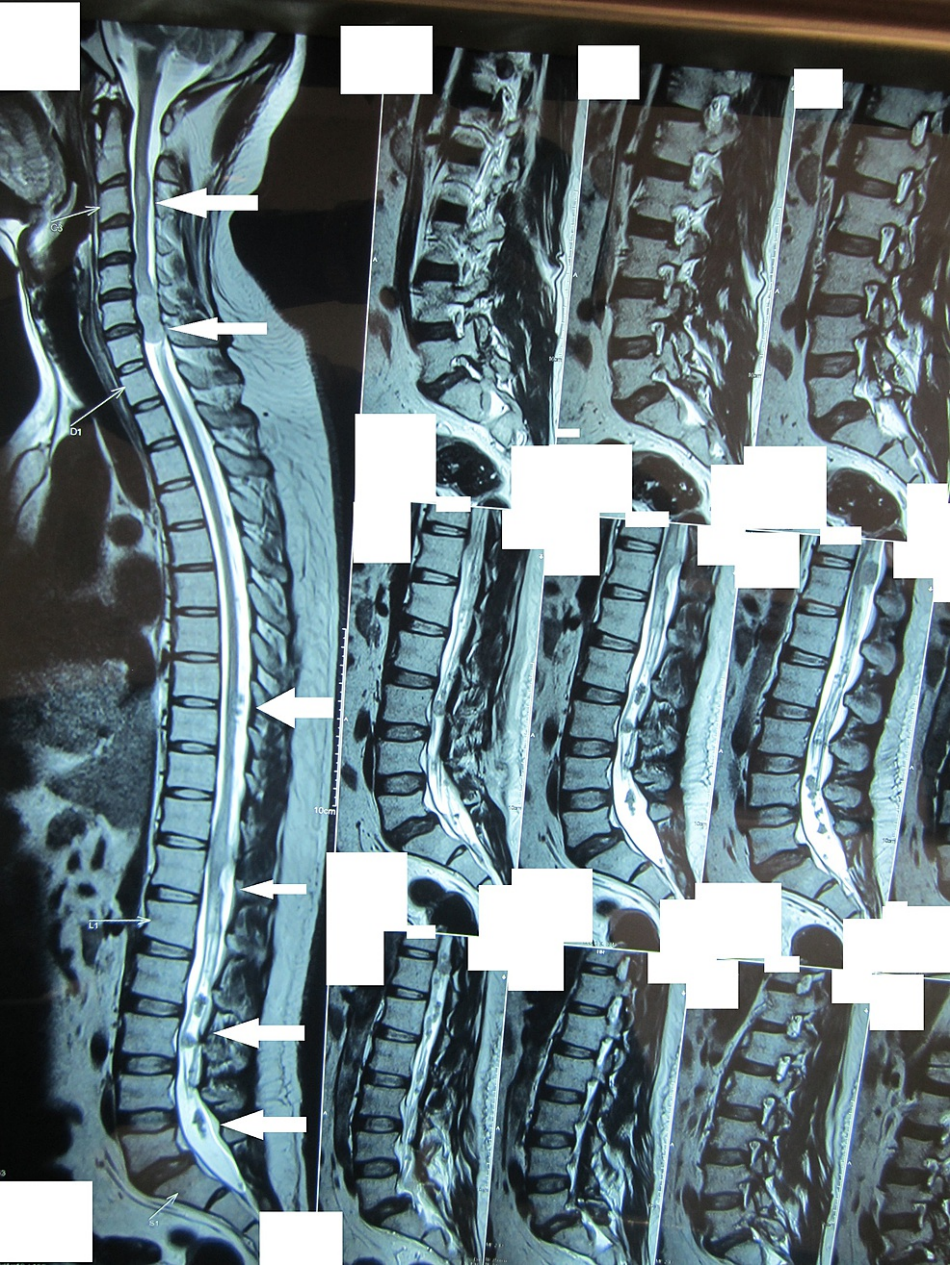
MRI sagittal section T2 sequence showing multiple tumors in different regions of the spinal cord (arrows) MRI: magnetic resonance imaging
